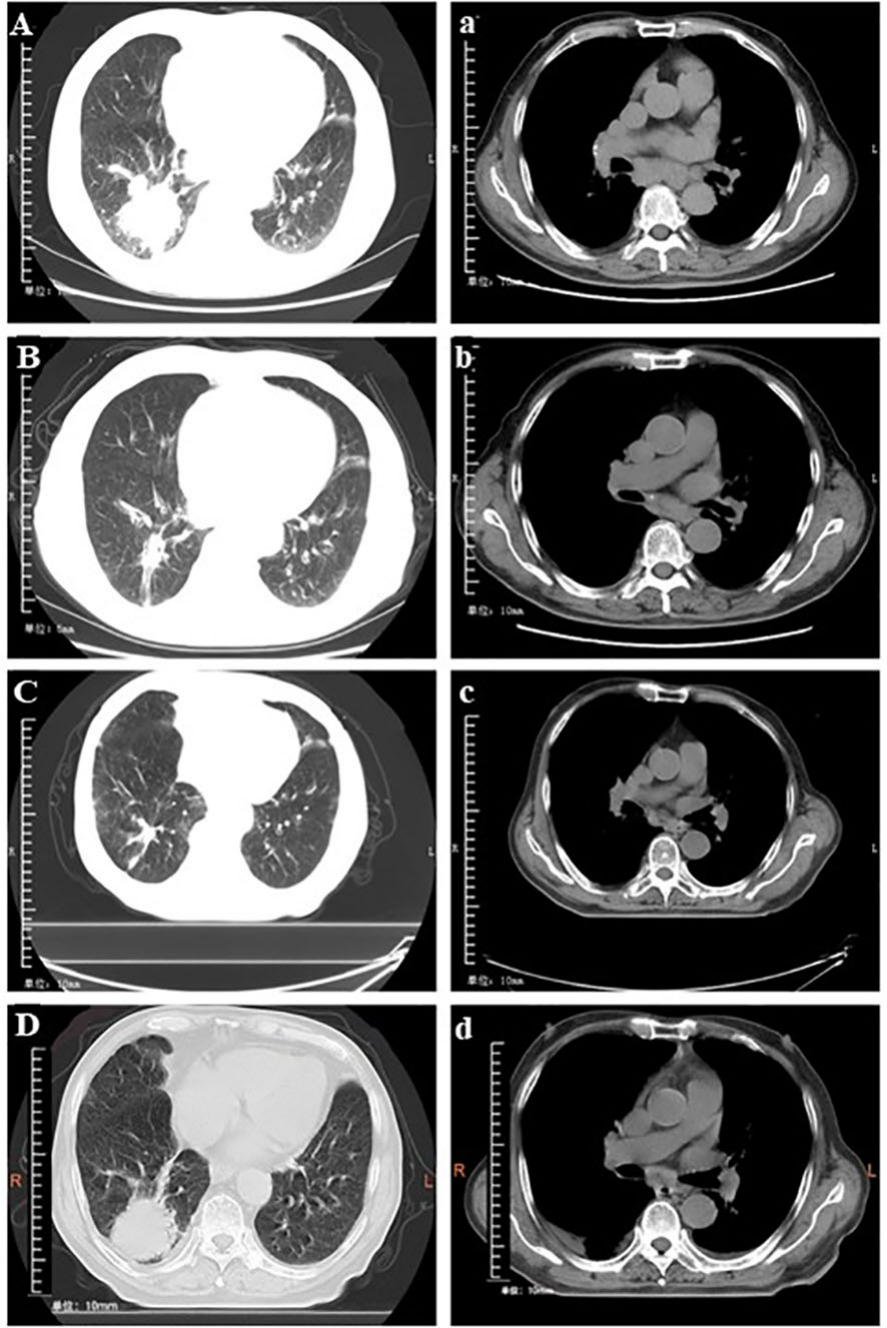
Conversion of chest computed tomography at different times. (A and a) Baseline findings on chest computed tomography (tumor size: 52 × 55 mm). (B and b) The mass shrank significantly (tumor size: 31 × 18 mm) after treatment with four cycles of anlotinib plus cisplatin. (C and c) Stable disease (tumor size: 25 × 21 mm) following 12 cycles of anlotinib + tegafur. (D and d) Progressive disease (tumor size: 41 × 34 mm) after 29 cycles of anlotinib + tegafur.
